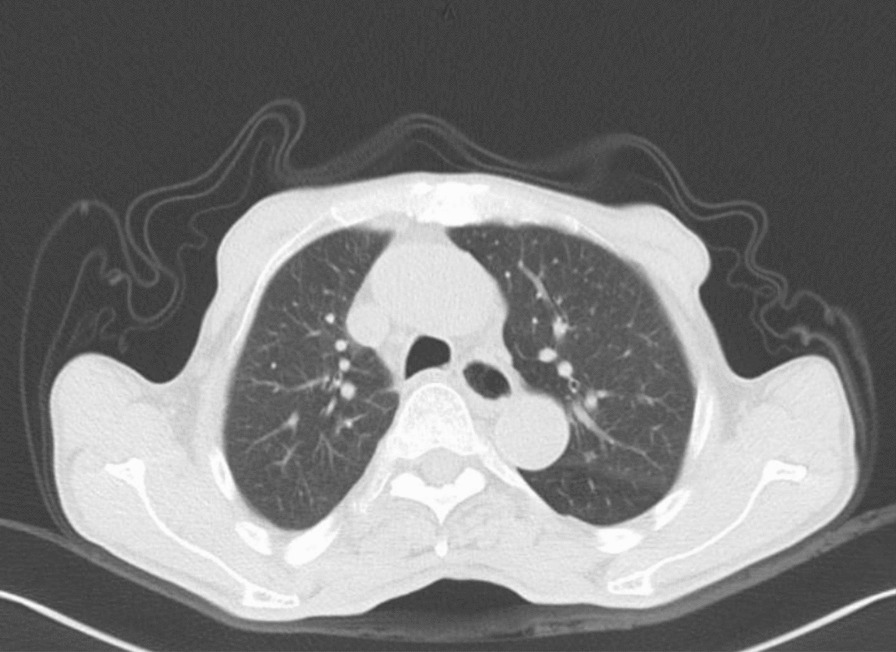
Chest CT at patient follow-up
